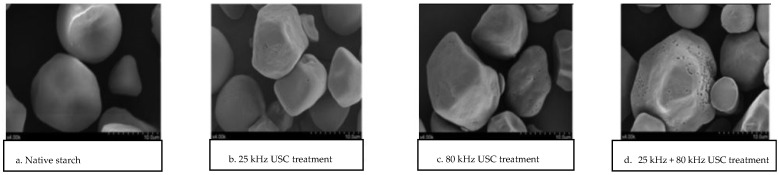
SEM photos of sweet potato starch samples sonicated for 60 min at different frequencies (4000×). Untreated starch (a), ultrasonicated at 25 kHz (b), ultrasonicated at 80 kHz (c), double USC treatment at 25 kHz and 80 kHz (d) [8].
